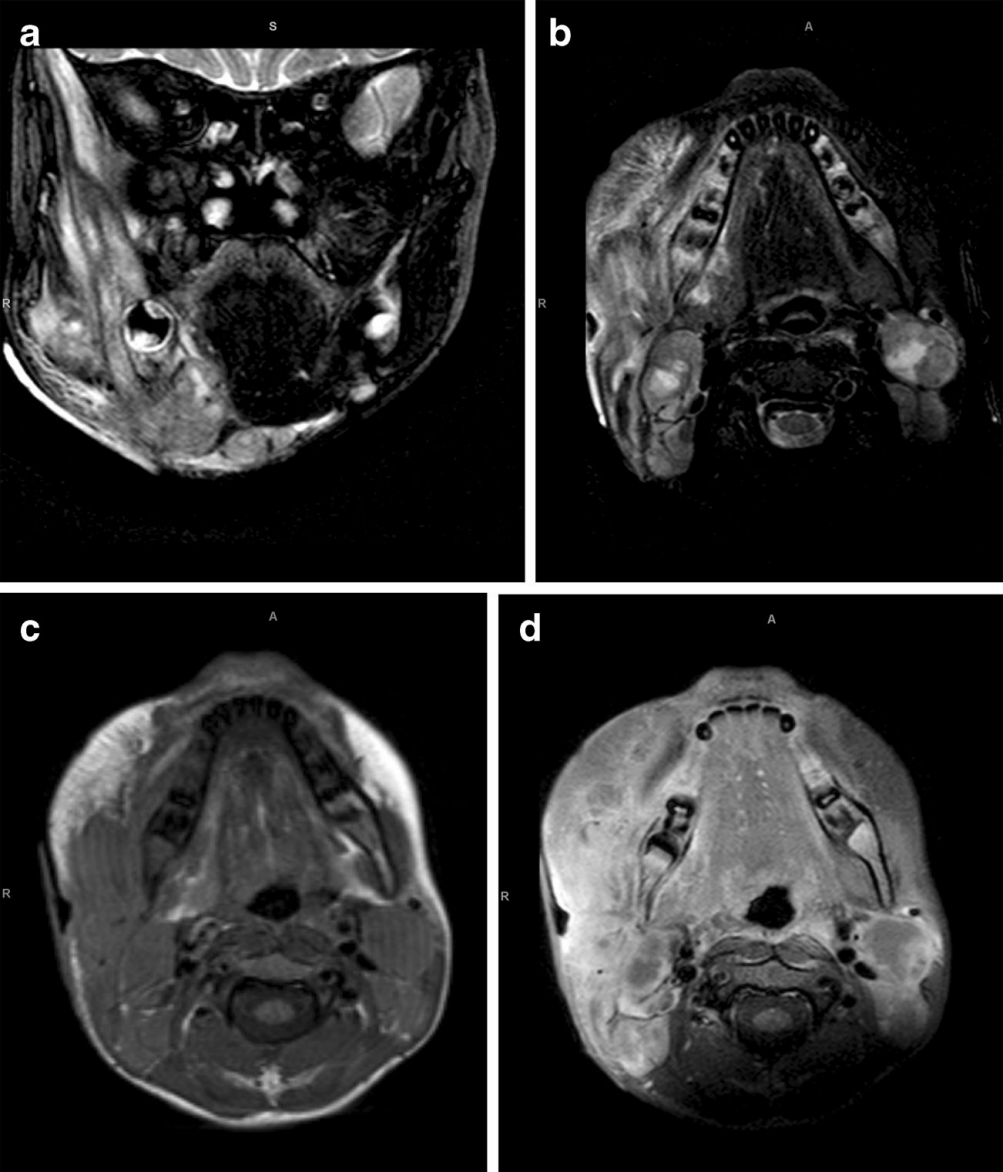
TB mandible. Coronal (a) and axial (b) T2-W MRI images show a destructive right mandibular lesion involving the ramus and coracoid process, with a periosteal reaction and associated soft tissue mass. The pre- (c) and post-contrast (d) T1-W images demonstrate heterogeneous enhancement of the soft tissue component. Characteristic of TB are large bilateral carotid space nodes which are predominantly T2 hypointense (b), with rim enhancement and central necrosis (d)
